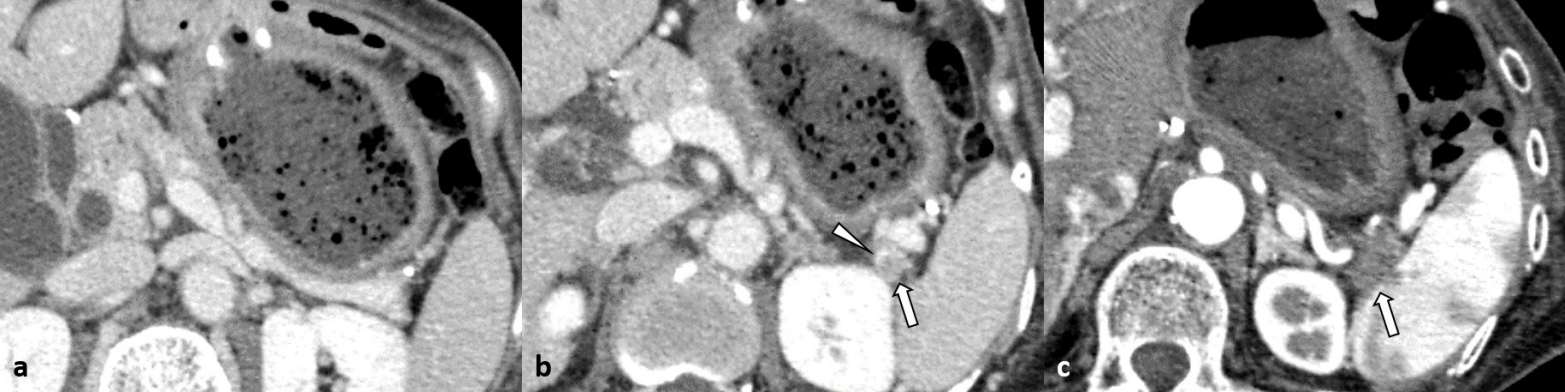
A 64-year-old female with pancreatic adenocarcinoma that developed after treatment for gastric cancer (Case 3) (a) Venous phase contrast-enhanced CT image obtained 26 months before the pancreatic surgery showing no abnormal findings suggestive of pancreatic adenocarcinoma. (b) Contrast-enhanced CT image obtained 14 months before the pancreatic surgery showing a small (8 mm diameter) hypoattenuation (arrow) lesion with an enhanced rim (arrowhead). (c) Triple-phase contrast-enhanced pancreatic phase CT image obtained immediately before pancreatic surgery showing an ill-defined hypoattenuated pancreatic tail mass (10 mm diameter, arrow). The pancreatic tail mass is invading the peripancreatic fat tissue and splenic hilum.
